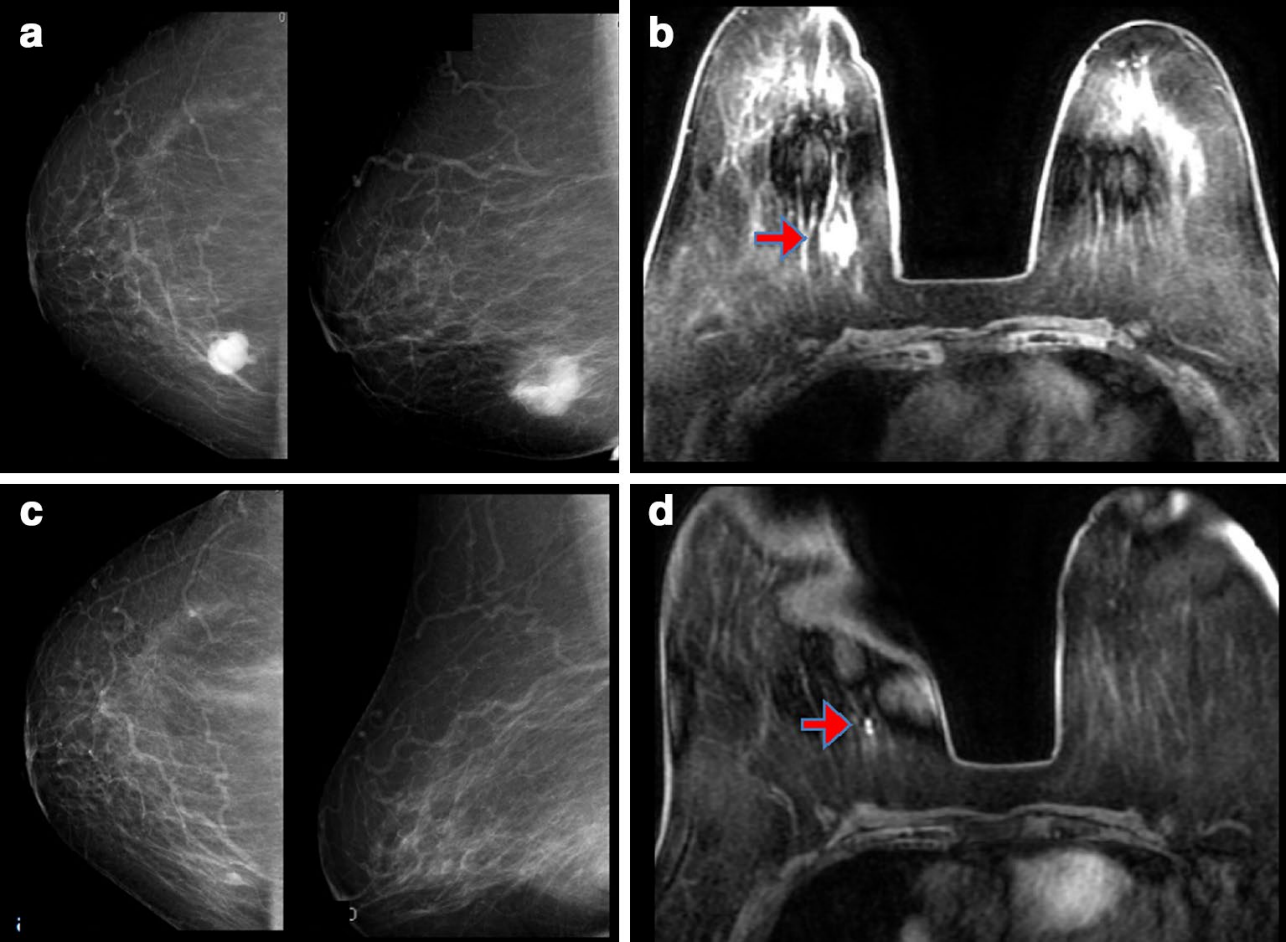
Radiologic appearance of the tumor. a Mammography shows a dense mass with partially indistinct margin in the right breast. b Contrast-enhanced MRI shows a high-intensity lesion on T2- and diffusion-weighted imaging. c, d After 12 months of letrozole treatment, mammography reveals disappearance of the tumor (c) and MRI shows no evidence of tumor (d)
